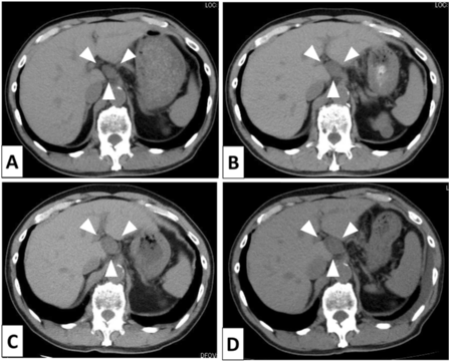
The CT scans of the past 3 years. (A) March, 2011. (B) March, 2012. (C) April, 2013. (D) January, 2014.
